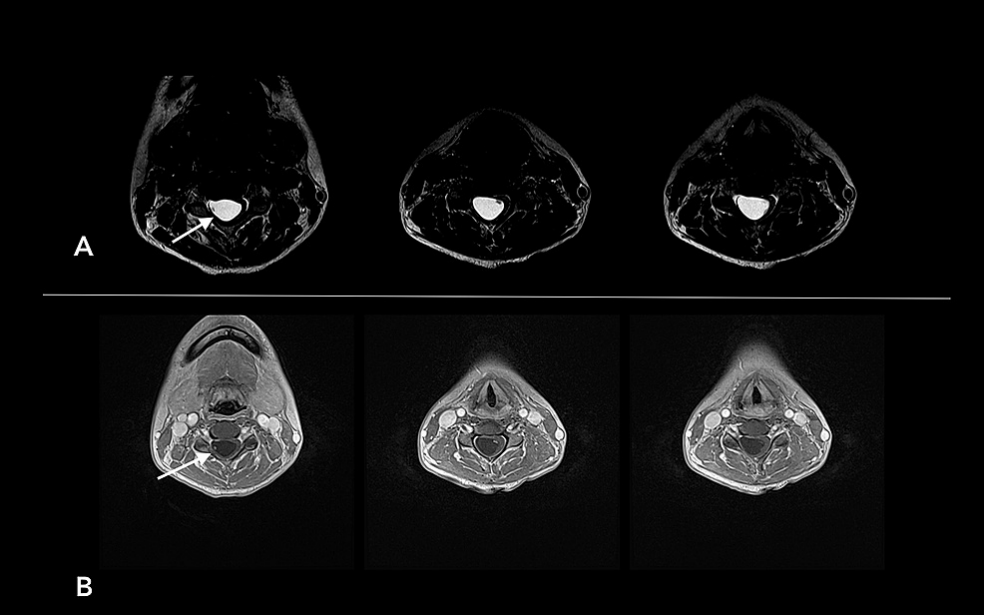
Axial MRI shows spinal intramedullary mass that appears hyperintense on T2 (arrow) (A) and hypointense on T1 (B)
